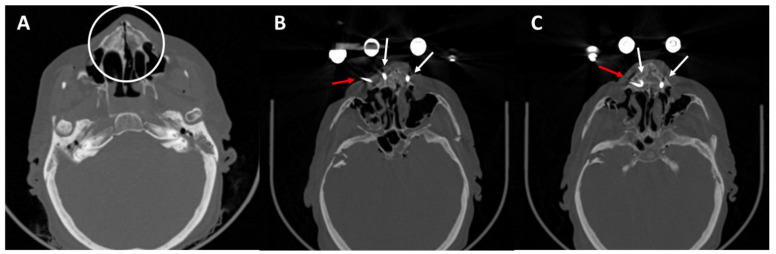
CT scans of the fractured skull (A) and palatal split (white circle). (B,C) Reduced palatal split. K-wire (red arrow) and two medial palatal pins (white arrows).
